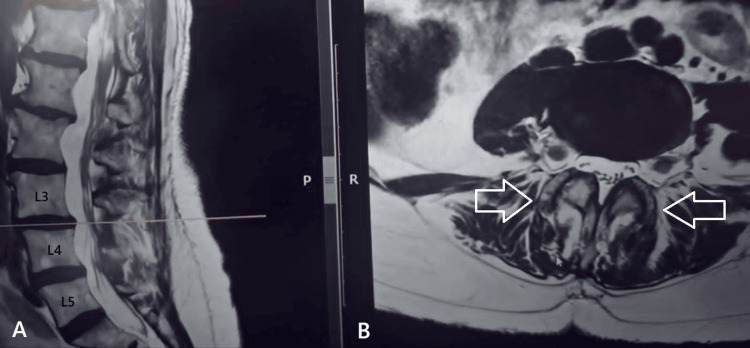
(A) T2-sagittal view and (B) T2-axial MRI views of the lumbar spine in first case. At the L3-L4 level, the arrows with the white outline indicate the hypertrophic facets (Grade 4 according to Grogan) with accompanying bilateral facet subluxation.
